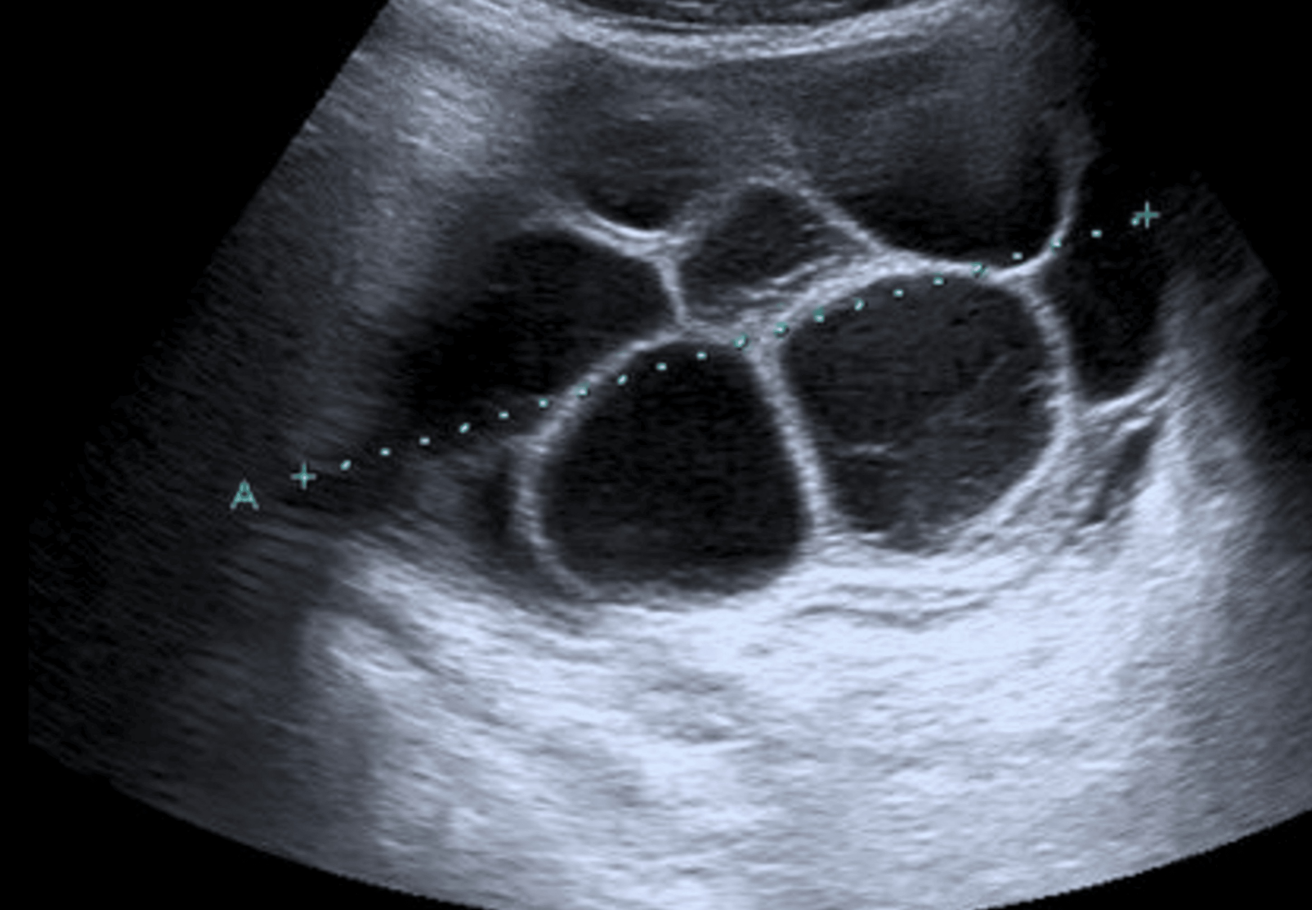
Pelvic ultrasound demonstrating bilateral enlarged multicystic ovaries with thin-walled cysts. Findings are characteristic of ovarian hyperstimulation syndrome in early pregnancy.
